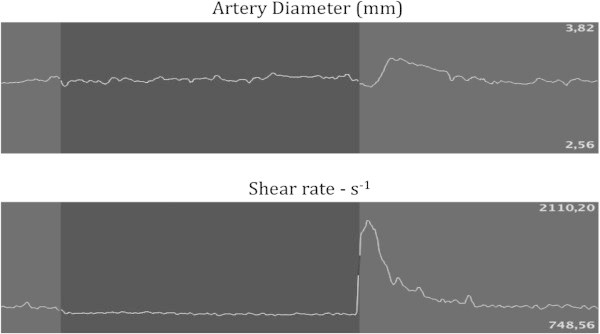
Flow-mediated dilation. Flow-mediated dilation (FMD) measures endothelial function by inducing a temporary ischemia in a brachial artery and observing the amount of vasodilation following the stressor event. After a baseline measurement of the artery diameter via an ultrasound probe, a sphygmomanometer blood pressure cuff is positioned on the right forearm 2 cm below the elbow and inflated to 250 mmHg to produce ischemia in the forearm. The cuff is deflated after some minutes, usually 5, thus causing a reactive hyperemia which in turn produces a shear stress stimulus that induces the endothelium to release nitric oxide as a vasodilator. FMD is considered as diameter after reactive hyperemia - basal diameter/basal diameter × 100. The figure shows the diameter (upper part) and shear rate (lower part) of brachial artery during FMD before (grey-shaded part on the left), during (black-shaded part), and after (grey-shaded part on the right) ischemia.
